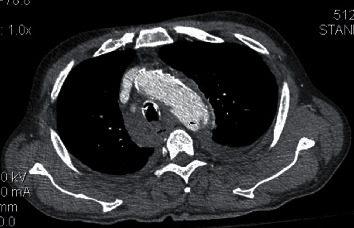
Preoperative CTA showed transection of the distal aortic arch.
